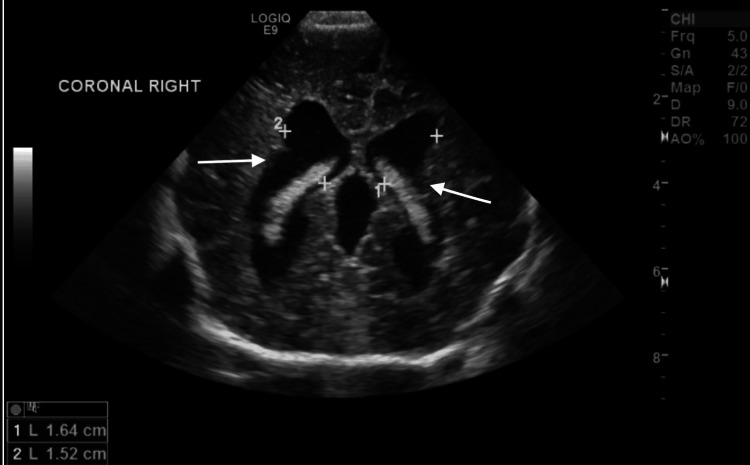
Ventriculomegaly of the lateral ventricles identified on ultrasound Ventriculomegaly of the lateral ventricles as indicated by the arrows
